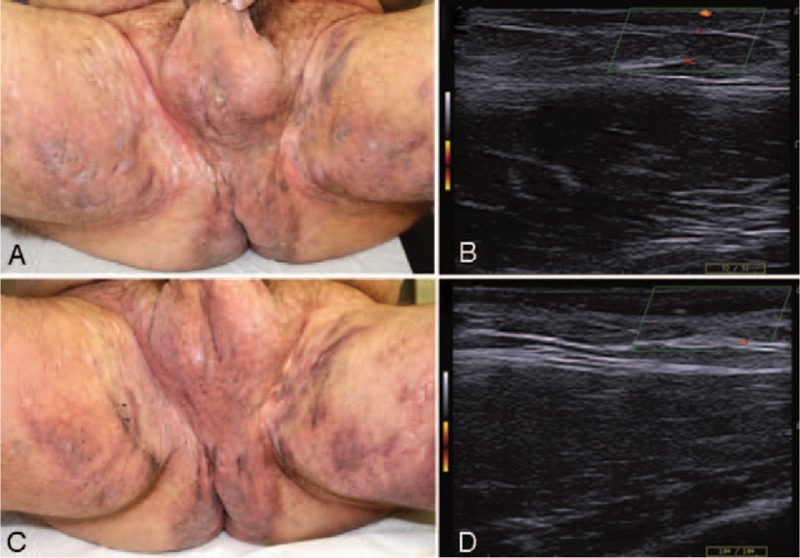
Case 2: (A) Very-severe Hurley III hidradenitis suppurativa (HS-PGA 5/5) with extensive involvement of the inguinal, perineal, perianal, and inner thigh areas. (B) Doppler ultrasound examination of left inguinal sinus-tract, at baseline. (C) Clinical improvement after 16 weeks of apremilast treatment. (D) Reduction of inflammation/color-flow signal, after 16 weeks of apremilast treatment.
